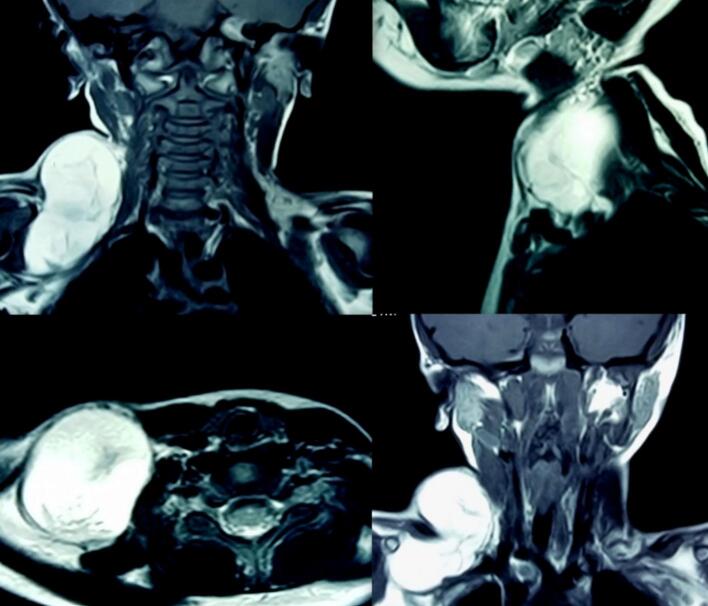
Plain MRI Findings of right supraclavicular lipoma.
